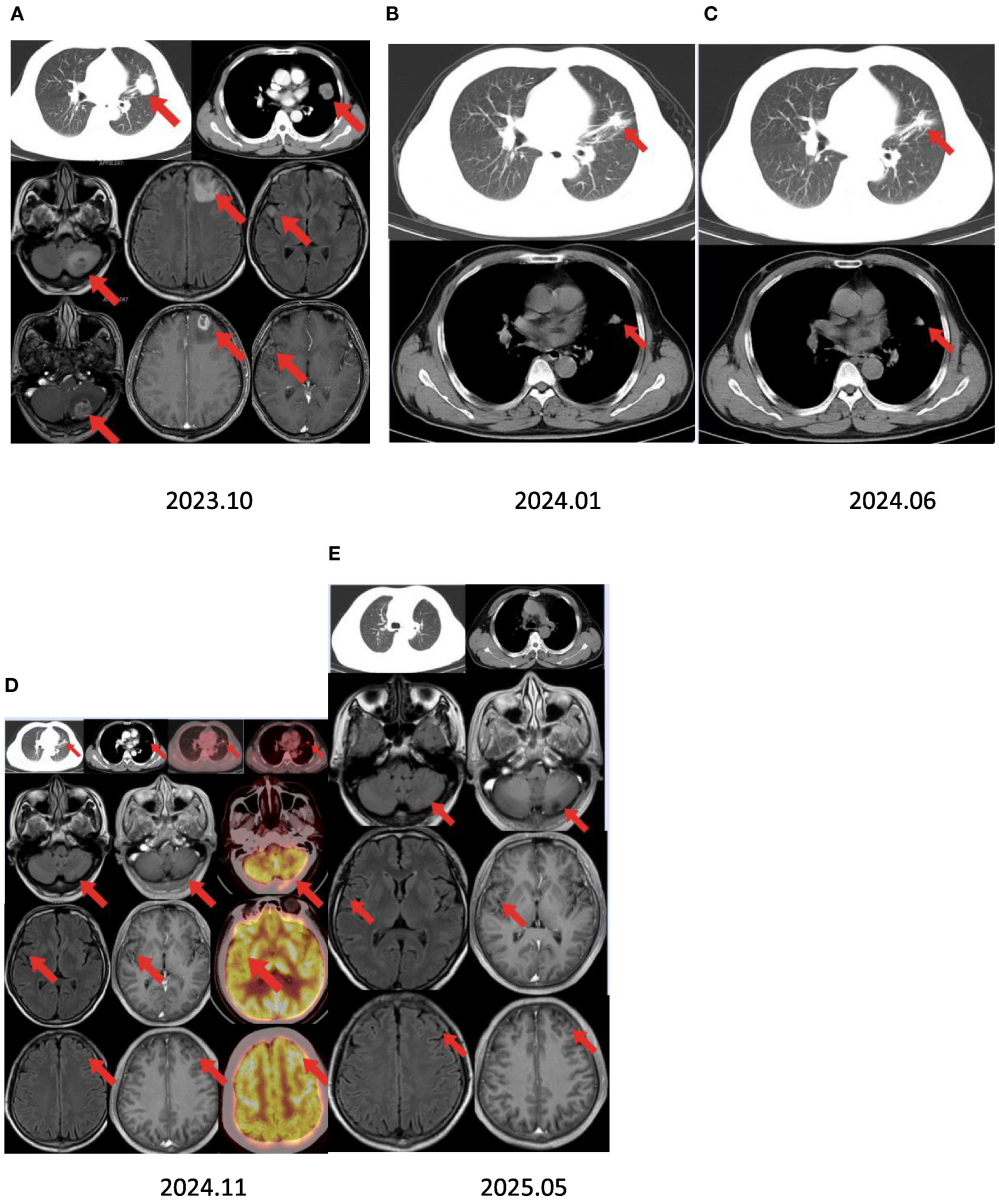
Timeline summary with dynamic imaging of the different therapeutic lines between October 2023 and May 2025. (A) Baseline chest CT showing a lung mass in the left upper lobe and brain MRI revealing multiple brain metastases. (B, C) Best response under chemotherapy and immunotherapy with a partial response after 2 and 7 months. Assessment showed a partial response of all lung lesions and complete response of the brain lesions after 11 months of furmonertinib. (D) Positron emission tomography/CT scan revealing a nodular soft tissue density shadow in the lingual segment of the left upper lobe and increased radioactive concentrations of foci, with a maximum standard uptake value (SUV_max_) of 3.8. There was no increased radioactive concentration of foci in the brain. (E) Brain MRI showing no recurrent lesions after 18 months.
